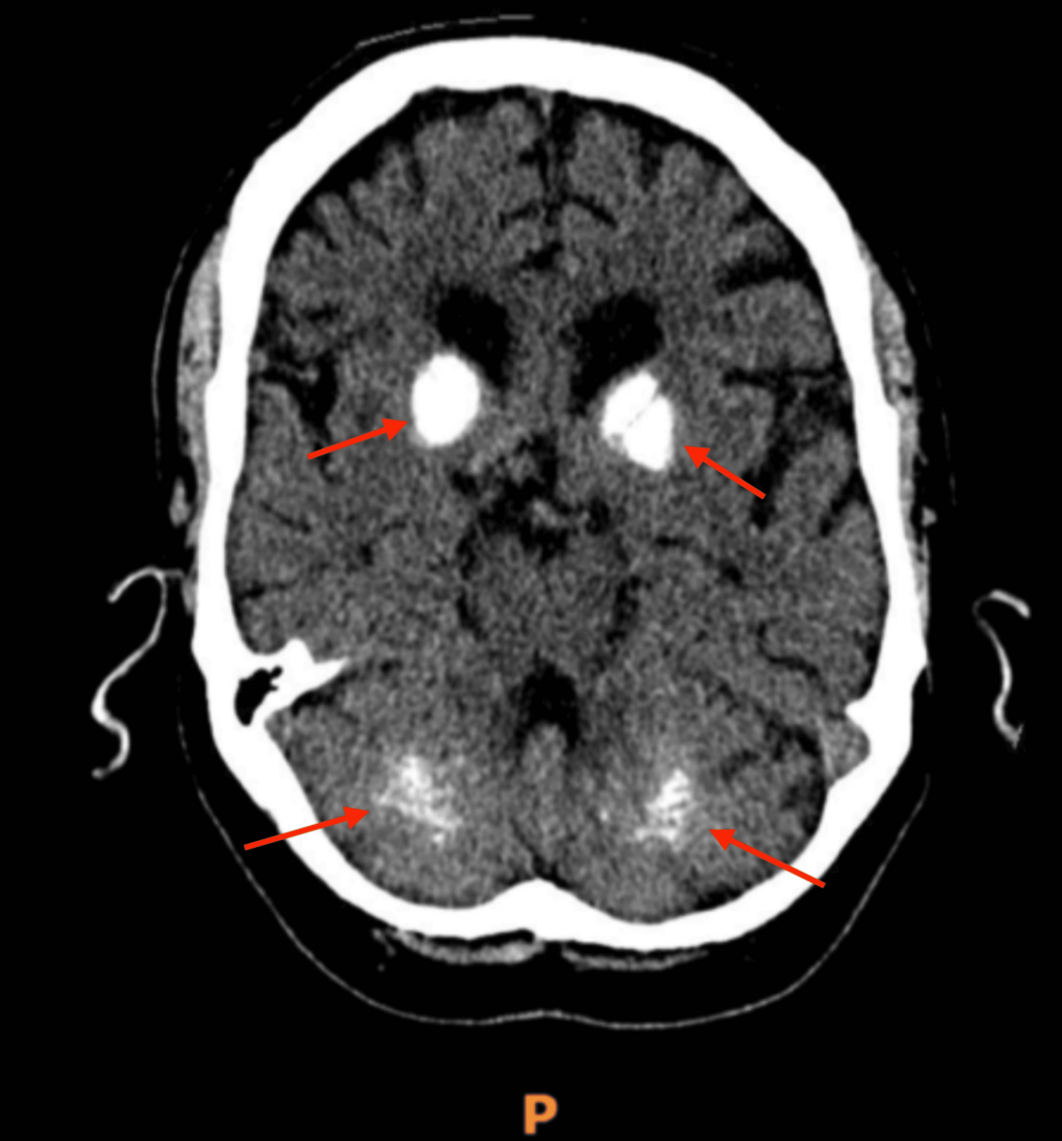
Axial non-contrast CT brain showing symmetric calcification of the bilateral basal ganglia and cerebellar dentate nuclei (arrows), consistent with Fahr’s syndrome.
